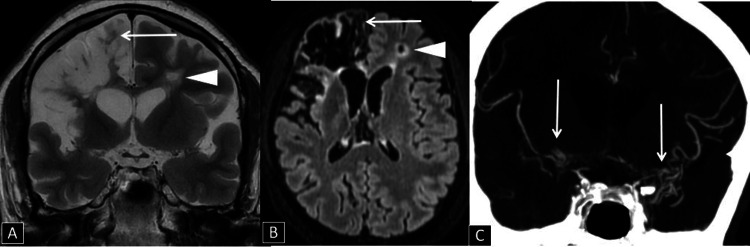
(A) Coronal T2WI image and (B) Axial T2 FLAIR image showing a large area of chronic infarct (arrow) involving the right frontal lobe, right insular region, and right-sided genu of the corpus callosum, and a small chronic infarct (arrowhead) in the left frontal white matter. (C) Coronal reformatted CT Angiogram of the brain showing occluded M1 and M2 segments of the bilateral MCAs replaced by multiple collaterals(arrow)  in the bilateral Sylvian cisterns(more on the left side). T2WI: T2 weighted image, FLAIR: Fluid-attenuated inversion recovery, MCA: Middle cerebral artery
